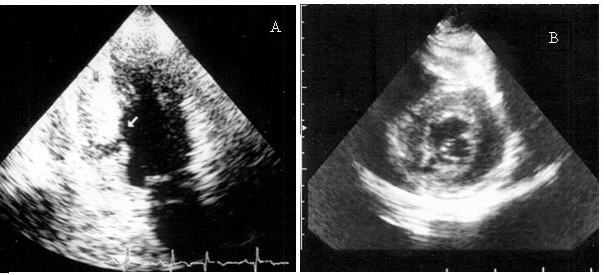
2D-Echo: apical two-chamber (A) and parasternal short axis (B) views showing a myocardial tear in the left ventricular inferior wall.
